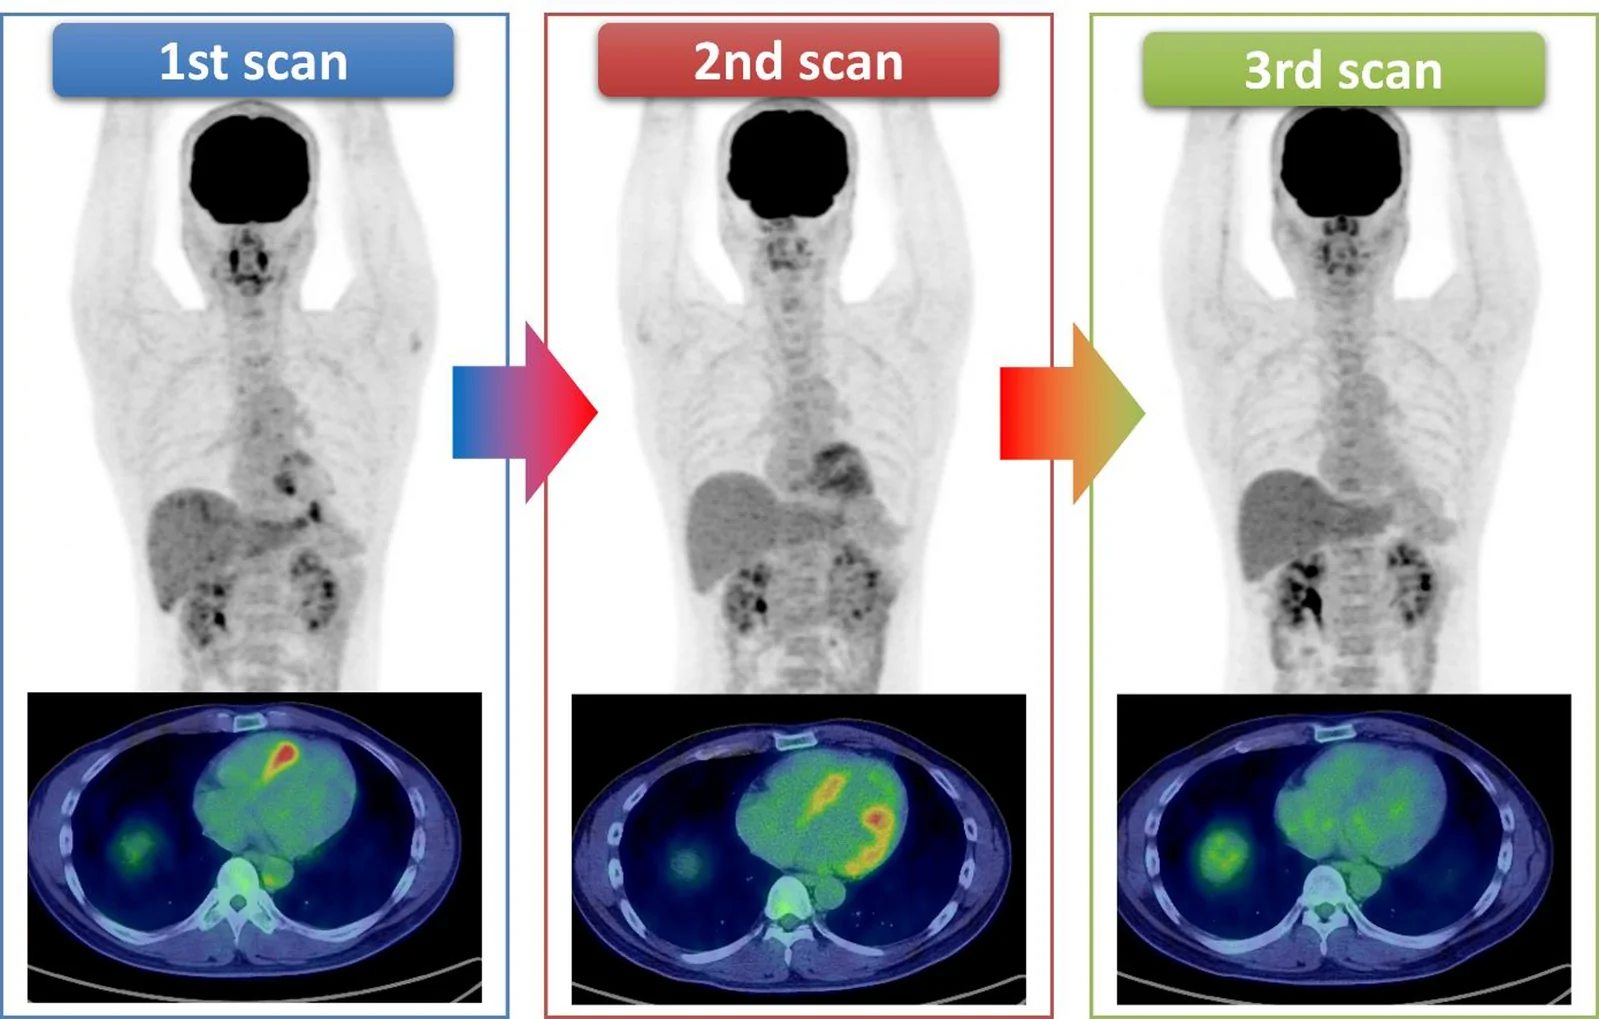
Serial changes in myocardial FDG uptake patterns in a representative patient with cardiac sarcoidosis. Whole-body maximum intensity projection (MIP) images (upper row) and corresponding axial fused PET/CT images (lower row) are shown. Before corticosteroid therapy (left), focal myocardial FDG uptake is observed. After initiation of prednisolone (PSL) at 30 mg/day (middle), the uptake pattern changes to predominantly basal/lateral uptake without diffuse background activity. During follow-up at a reduced dose of PSL (10 mg/day) (right), myocardial FDG uptake is completely suppressed. These temporal changes support the interpretation that the post-treatment uptake represents physiological myocardial FDG uptake rather than residual inflammatory activity
